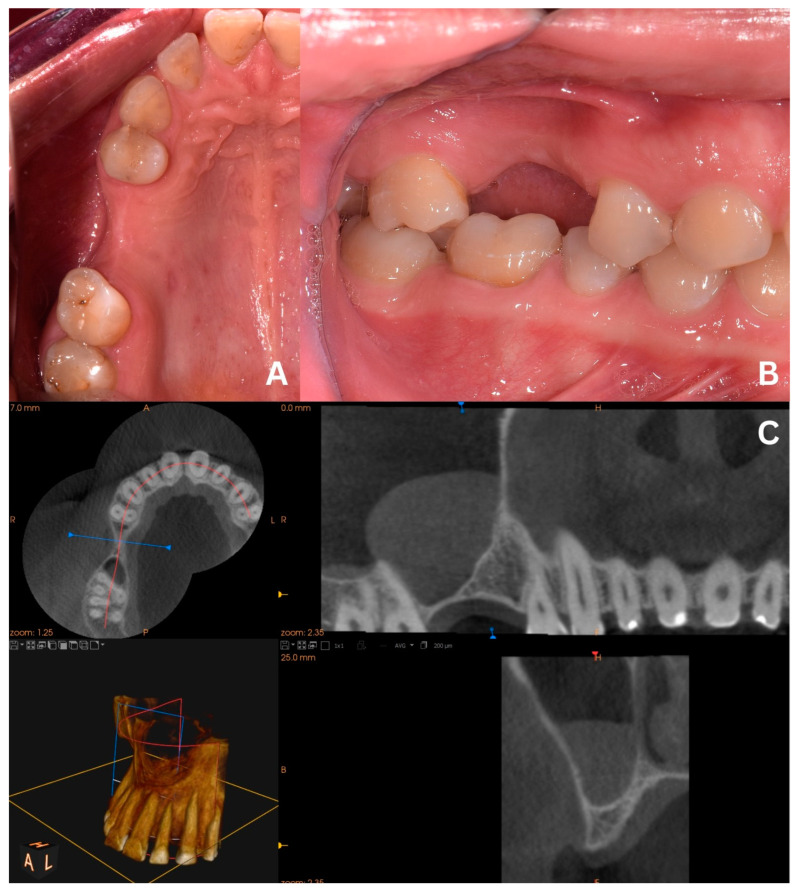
Clinical situation before the surgery. Occlusal view (A), lateral view (B), and CBCT scans with a dome-shaped radiopaque lesion in the sinus and insufficient bone height that requires sinus lift before placing implants (C).
